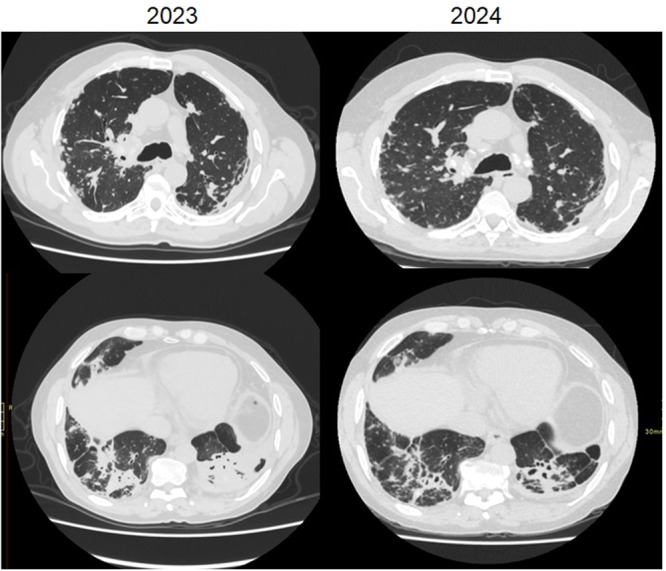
Silicosarcoidosis (Cases 4, 6, 9, and 12). Representative high‐resolution computed tomography (HRCT) images and clinical evolution of patients classified as *silicosarcoidosis*, showing mixed radiological features of both diseases—centrilobular and perilymphatic micronodules, fibrotic masses, and mediastinal lymph node calcifications. Three of four cases demonstrated radiological improvement after immunosuppressive therapy, particularly Cases 4 and 6, consistent with a partial inflammatory component.
